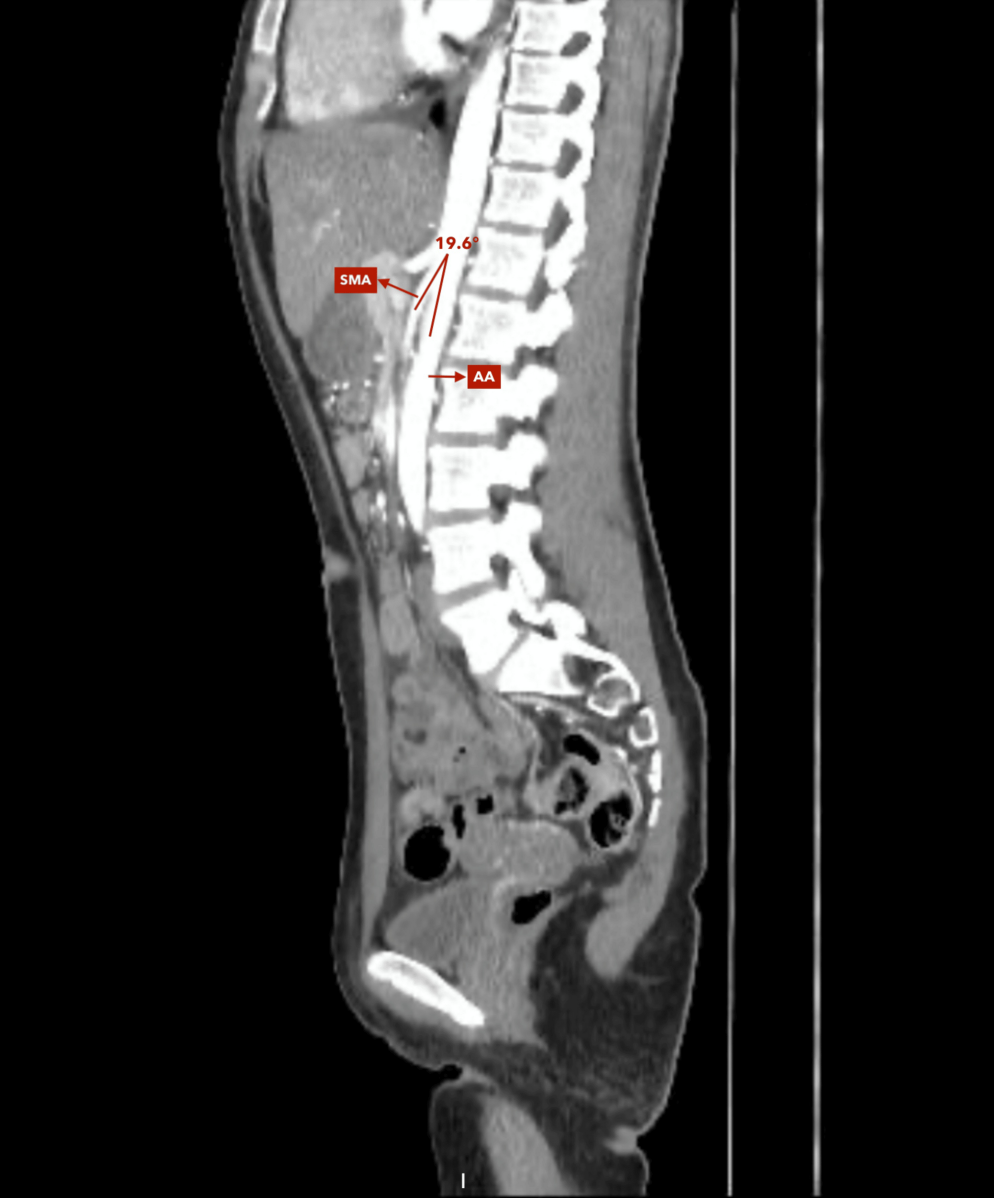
Contrast-enhanced abdominal CT scan (sagittal reconstruction, arterial phase) Approach for measuring the aorto-mesenteric angle. Angle between the abdominal aorta (AA) and superior mesenteric artery (SMA) of 19.6°.
